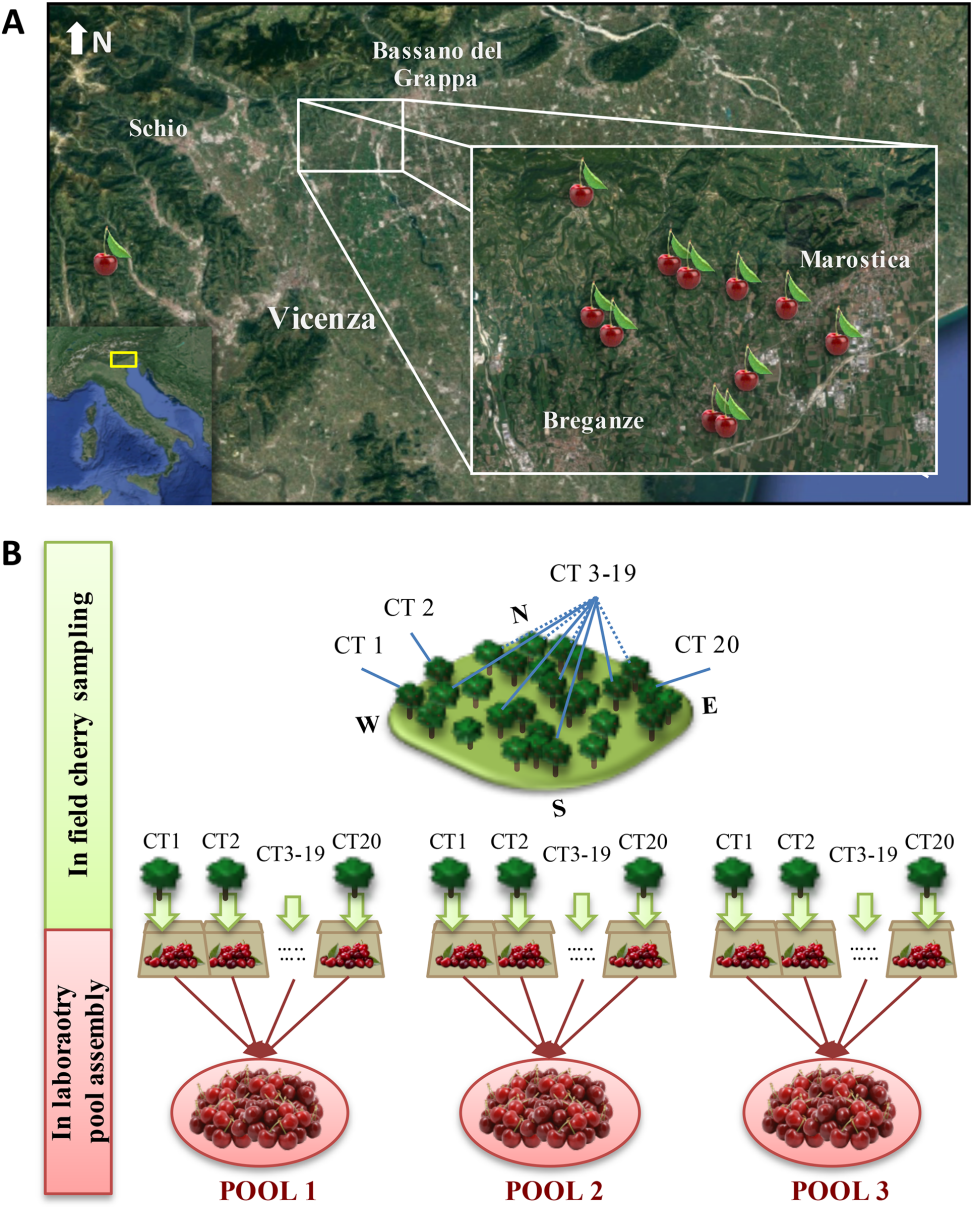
Sweet cherry sampling plan. A) The Vicenza region in Italy, showing the locations of the 12 different farms as cherry icons. B) The two-step sampling plan: field sampling by collecting cherries from 20 trees, and laboratory sampling by assembling three pools of 50 cherries. CT = cherry tree.
